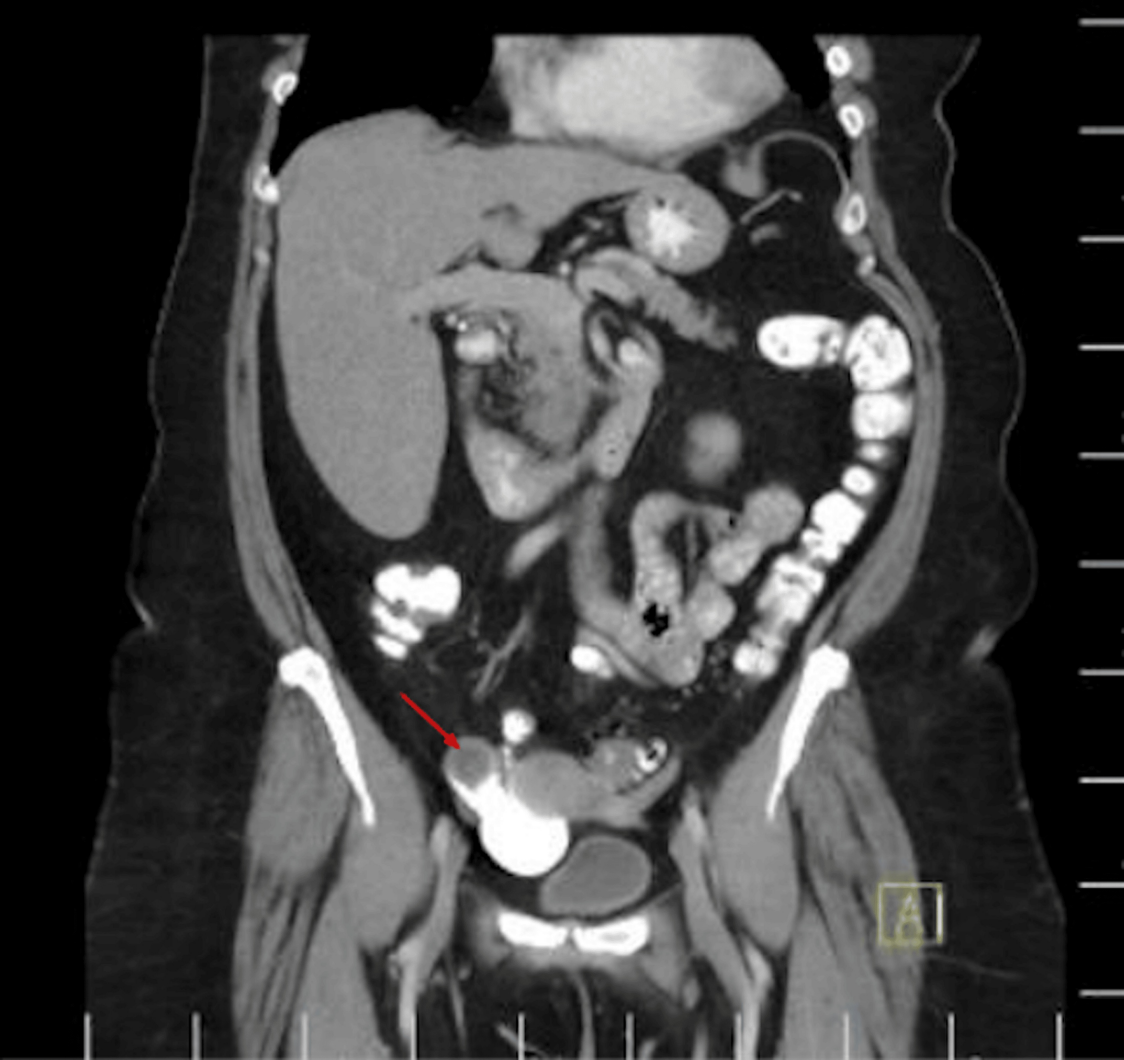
Coronal CT of the abdomen and pelvis; the red arrow demonstrates an oval cystic mass in the right lower quadrant with circumferential wall enhancement (post-colonoscopy, 2024).
